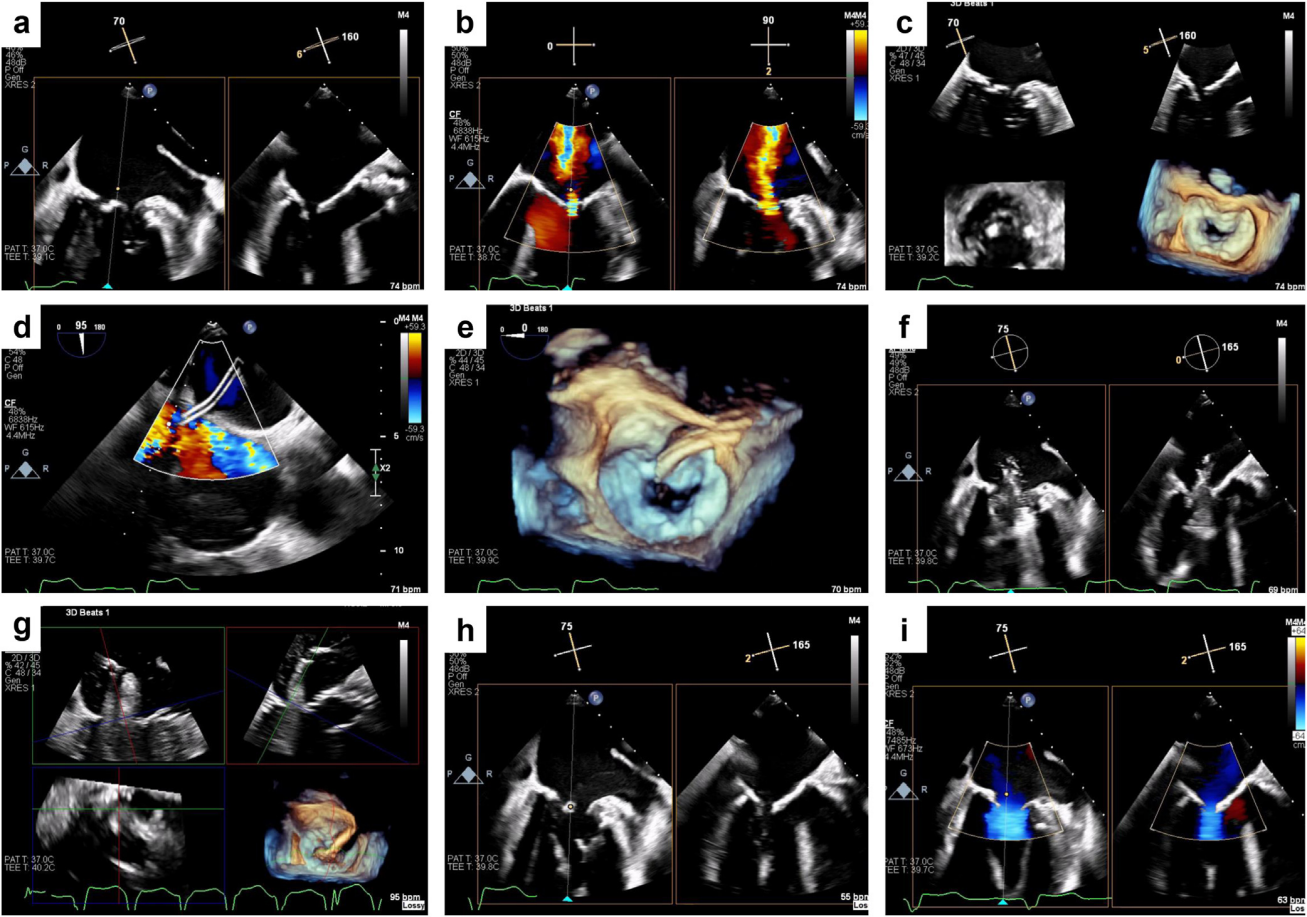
Representative transesophageal echocardiographic images of the MATRIX procedure. (a) Severe MS preprocedure on 2D TEE. (b) Severe MS preprocedure on 2D color Doppler. (c) Severe MS preprocedure on 3D reconstruction. (d) Transseptal crossing. (e) Alignment of the PASCAL precision system with the orifice of the MV. (f) MATRIX on 2D TTE. (g) MATRIX on 3D echo. (h) Post-MATRIX moderate MS on 2D echo. (i) Post-MATRIX moderate MS on 2D color Doppler. Abbreviations: 2D, two-dimensional; 3D, three-dimensional; MATRIX, Mitral Annulo-TRIpsy in eXtreme risk patients; MS, mitral stenosis; MV, mitral valve; TEE, transesophageal echocardiogram; TTE, transthoracic echocardiogram.
